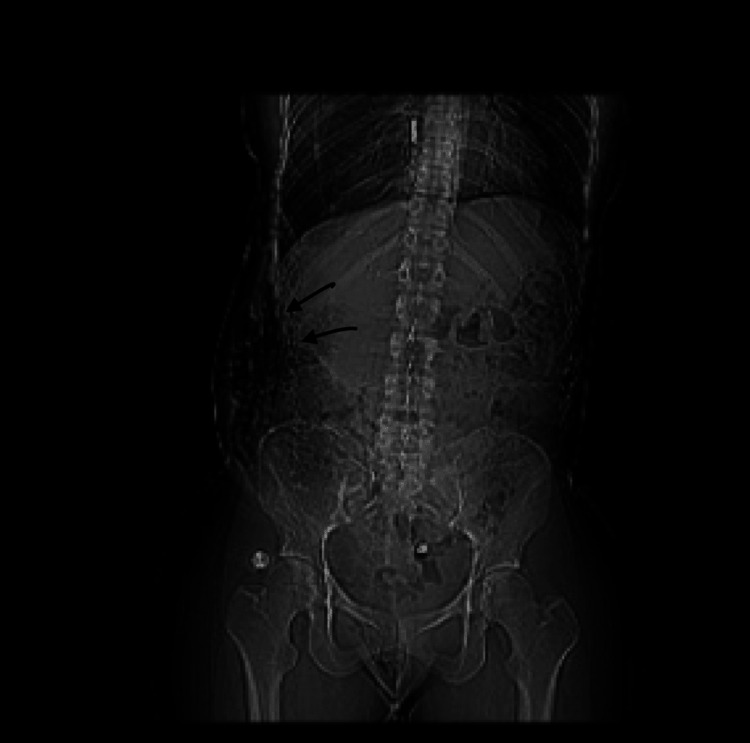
A CT of the patient's abdomen and pelvis with contrast demonstrates some ascites in the pelvis and confirms a large right-side pleural effusion with a central shift to the left side and at least two-thirds of right lung atelectasis. Compression atelectasis is visualized in the left lung.
